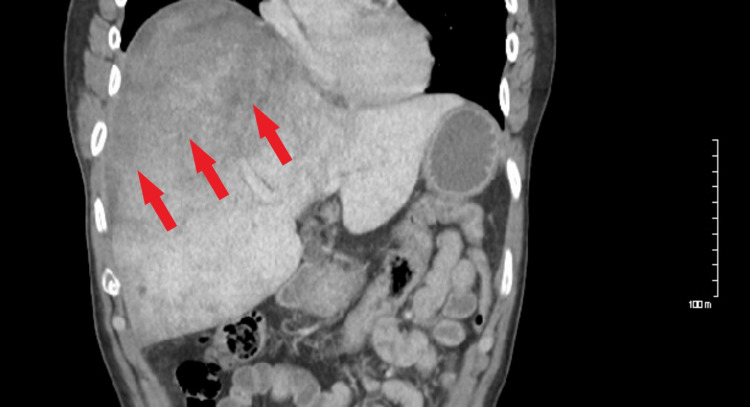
A CT scan shows spontaneous hepatic hemorrhage
